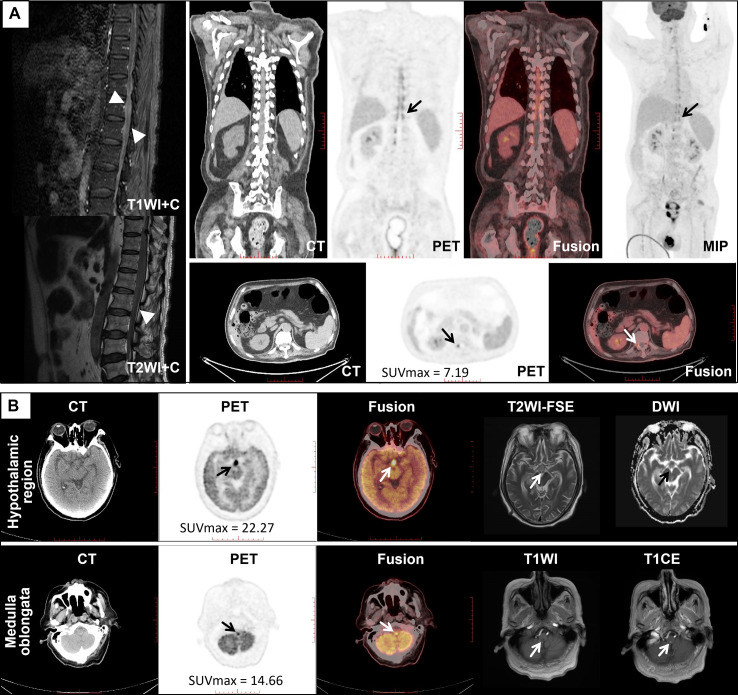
Imaging features of GBS with nervous system involvement. (A) Typical polyradiculitis: Enhanced MRI shows abnormal enhanced foci within the spinal canal at the T11-L2 level, associated with spinal cord compression and edema (white triangles). PET/CT fusion images demonstrate increased FDG uptake in the nerve roots of the cervical, thoracic, and lumbar segments of the spinal cord (arrows); (B) atypical CNS involvement: multiple diffuse and heterogeneous areas of increased FDG metabolism are observed on the surface of the cerebral cortex, cerebellum, hypothalamic region (upper row, arrows), and dorsal medulla oblongata (lower row, arrows), indicating a widespread inflammatory process extending beyond the typical lesion range. The enhanced MRI of the aforementioned area shows abnormal signals (arrows).
